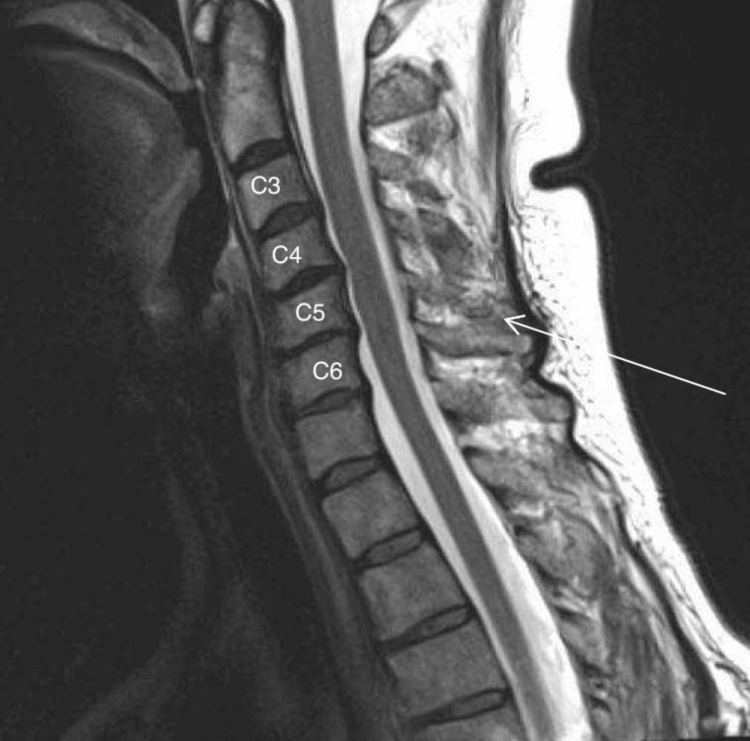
Cervical MRI showing foraminal stenosis (arrow) at the C5-C6 level MRI: magnetic resonance imaging
